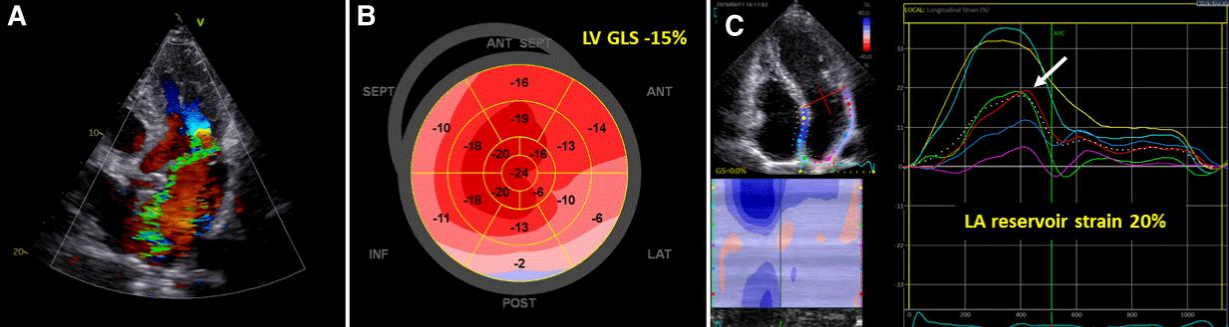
(*A*) Assessment of left ventricular global longitudinal strain and left atrial reservoir strain by speckle tracking echocardiography, in a patient with mitral valve prolapse and severe mitral regurgitation. (*B*) A bull’s eye plot displays the regional longitudinal strain for each left ventricular segment with a colour code; in this patient, global longitudinal strain (average of the 17 segments) was significantly impaired (being left ventricular global longitudinal strain value considered normal between −18 and –20%). (*C*) The longitudinal strain curve of the left atrial derived from the apical 4 chamber view (dotted line as average of the segments), and the peak positive strain is the left atrial reservoir strain, which was also impaired in this patient (as <22%).
